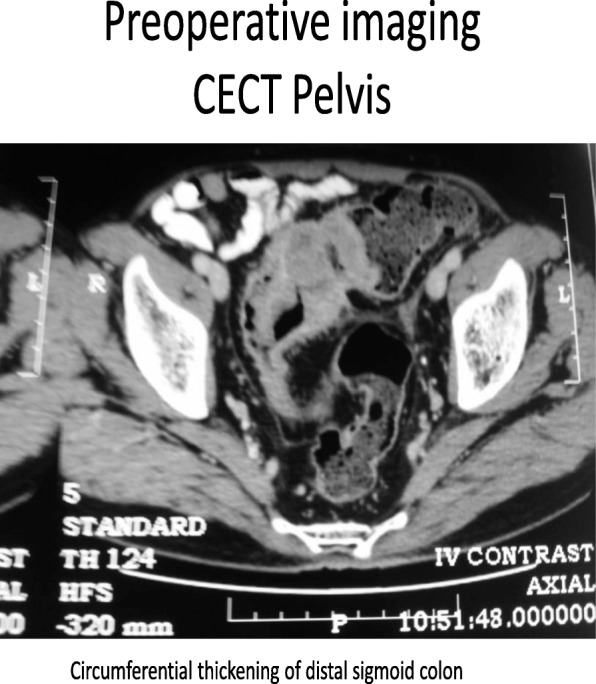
Circumferential thickening of sigmoid colon on contrast-enhanced computed tomography.
